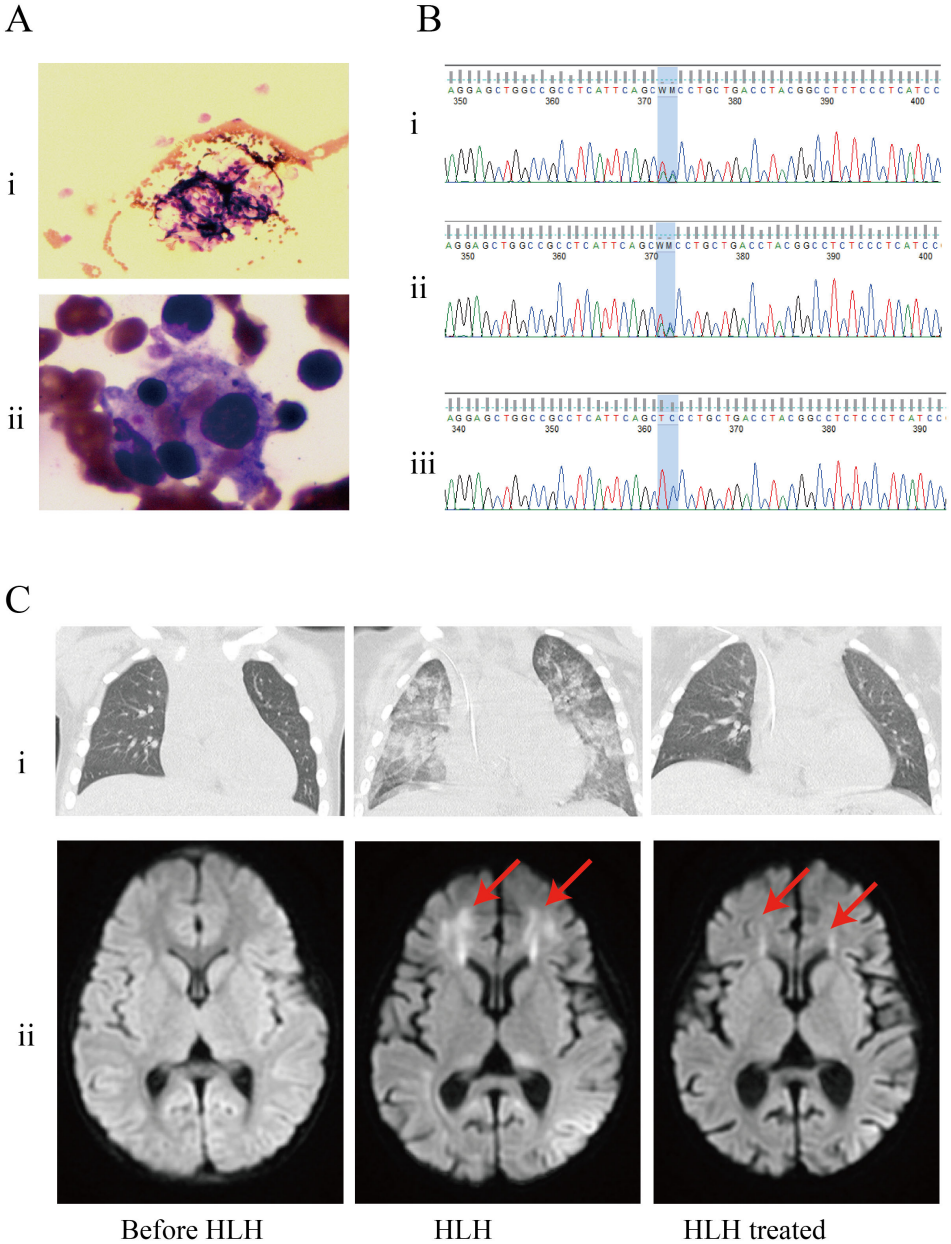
Patients examination results. (A) Bone marrow biopsy found histiocytes phagocytosing blood cells. (i) Histiocytes phagocytosed blood cells (arrow); (ii) Hemophagocytic cells underwent phagocytosis (arrow). (B) Results of whole-exome sequencing: the genetic analysis identified that the patient and her father had heterozygous mutations in UNC13D. (i) patient; (ii) father; (iii) mother. (C) The results of imaging examinations were as follows, (i) CT scan showed exudative lesions in both lungs; (ii) brain magnetic resonance imaging (MRI): a T2−weighted sequence showing abnormal white matter signal (arrow).
